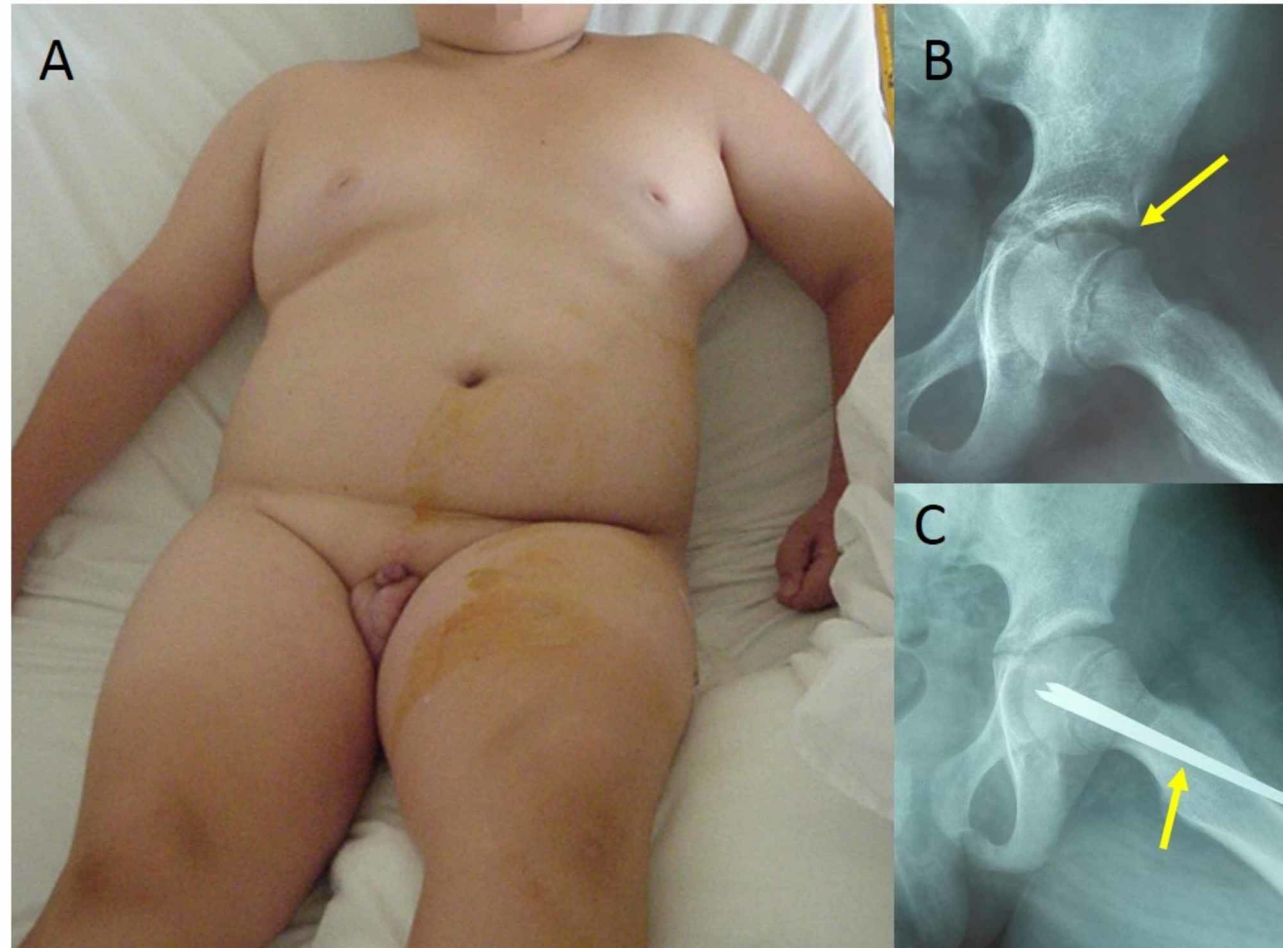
SCFE of the left hip in a 9-year-old obese boy with hypotrophic external genital organs (A) the adiposogenital phenotype: obesity combined with small external genital organs, (B) frog-lateral x-ray of the left hip indicates the SCFE (arrow), (C) in situ stabilization with two 6 mm Steinman pins (arrow) SCFE: slipped capital femoral epiphysis
